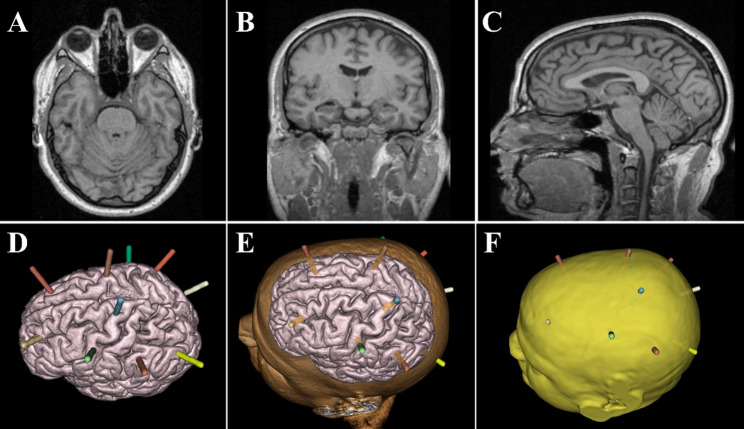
MRI-based brain segmentation and 3D reconstruction. A–C Cross-sectional, coronal, and sagittal views of cranial MRI. D–F MRI-based brain segmentation, TMS multi-target point marking, and 3D model creation
